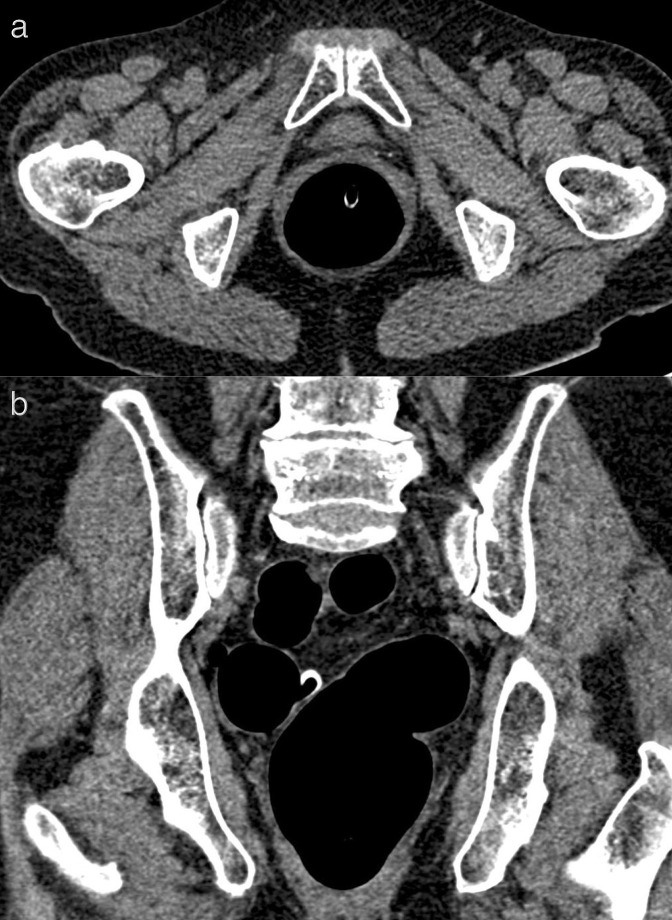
Axial (a) and coronal (b) image of virtual CT colonoscopy from 2016 showing no rectal or pelvic pathology.
